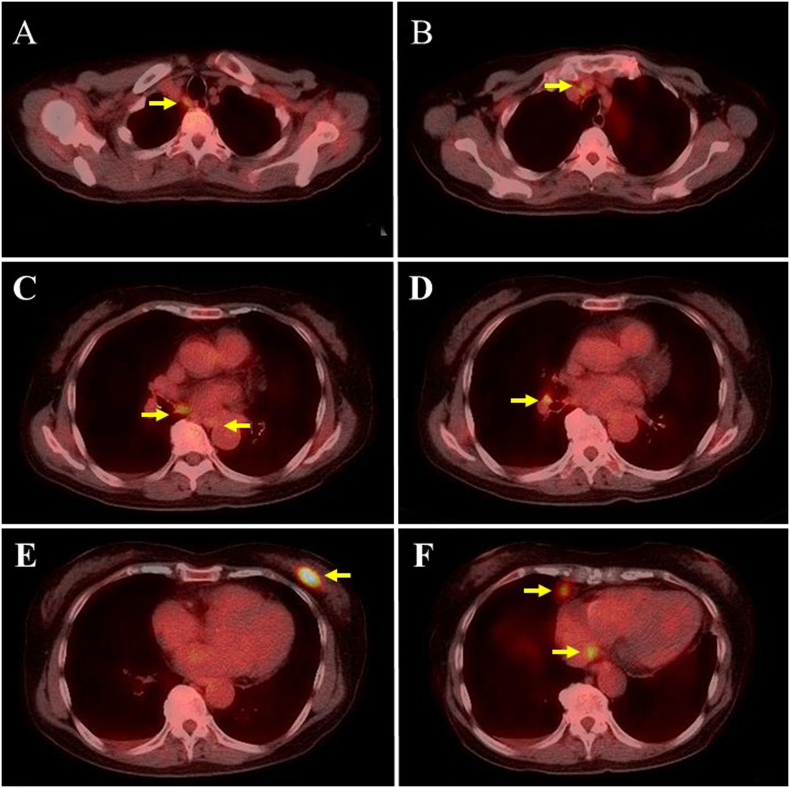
Abnormal accumulation during positron emission tomography-computed tomography Positron emission tomography-computed tomography images showing abnormal accumulations (yellow arrows) in the peritracheal lymph nodes (LNs) (A, C, and D), parasternal LNs (B and F), mediastinal LNs (C and F), breast tissue (E), and pericardial LNs (F) even though these LNs were small and not enlarged. (For interpretation of the references to colour in this figure legend, the reader is referred to the web version of this article.)
